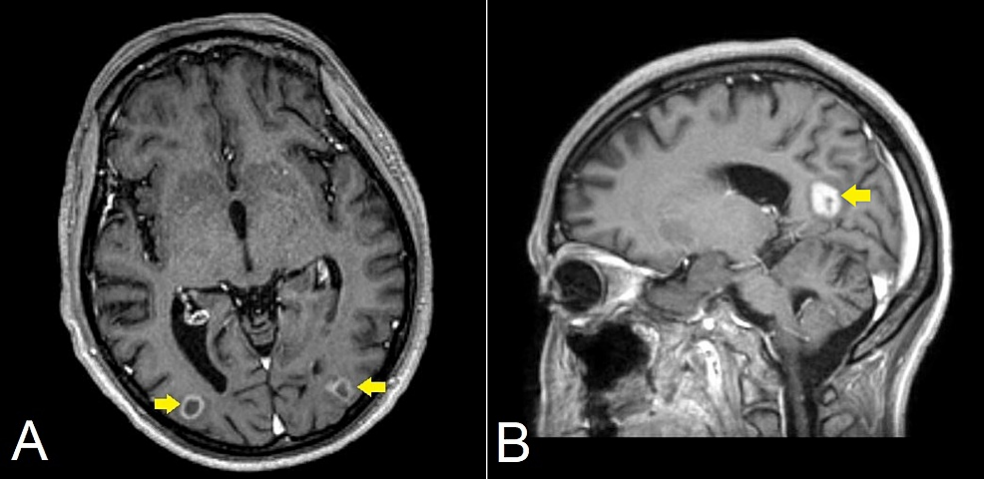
Axial (A) and sagittal (B) T1-weighed contrast-enhanced MRI images in a patient with PCNSL The images show multiple contrast-enhanced ring-enhancing lesions (yellow arrows) within the brain parenchyma with adjacent vasogenic edema MRI: magnetic resonance imaging; PCNSL: primary central nervous system lymphoma
